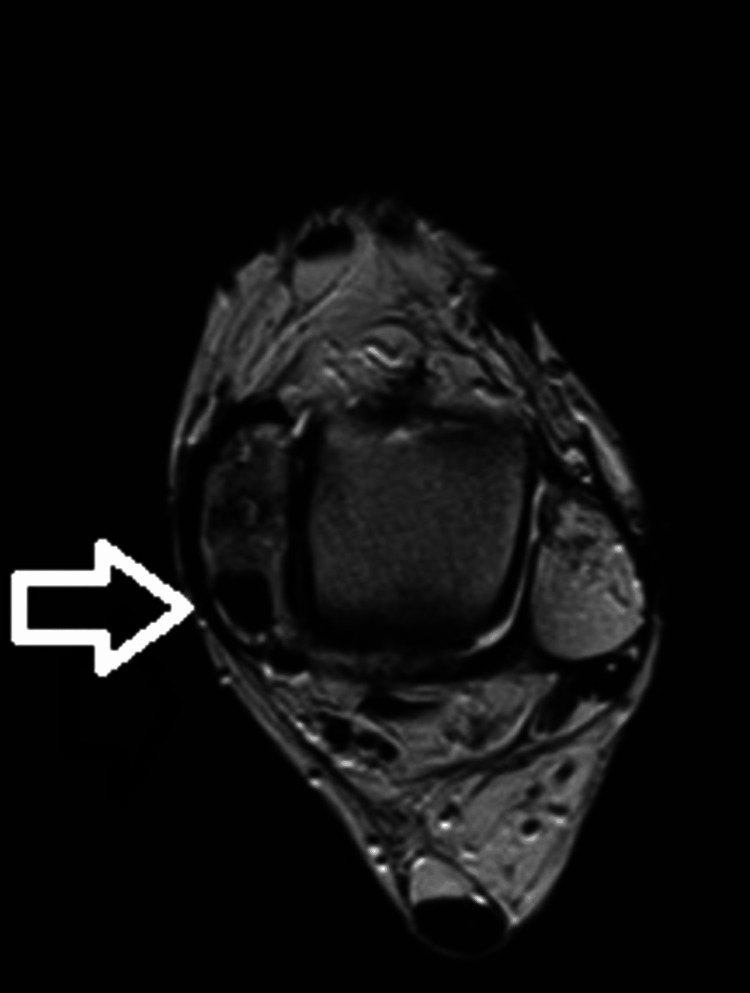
MRI scan of the ankle showing the formation of the osseous tunnel in the medial malleolus
